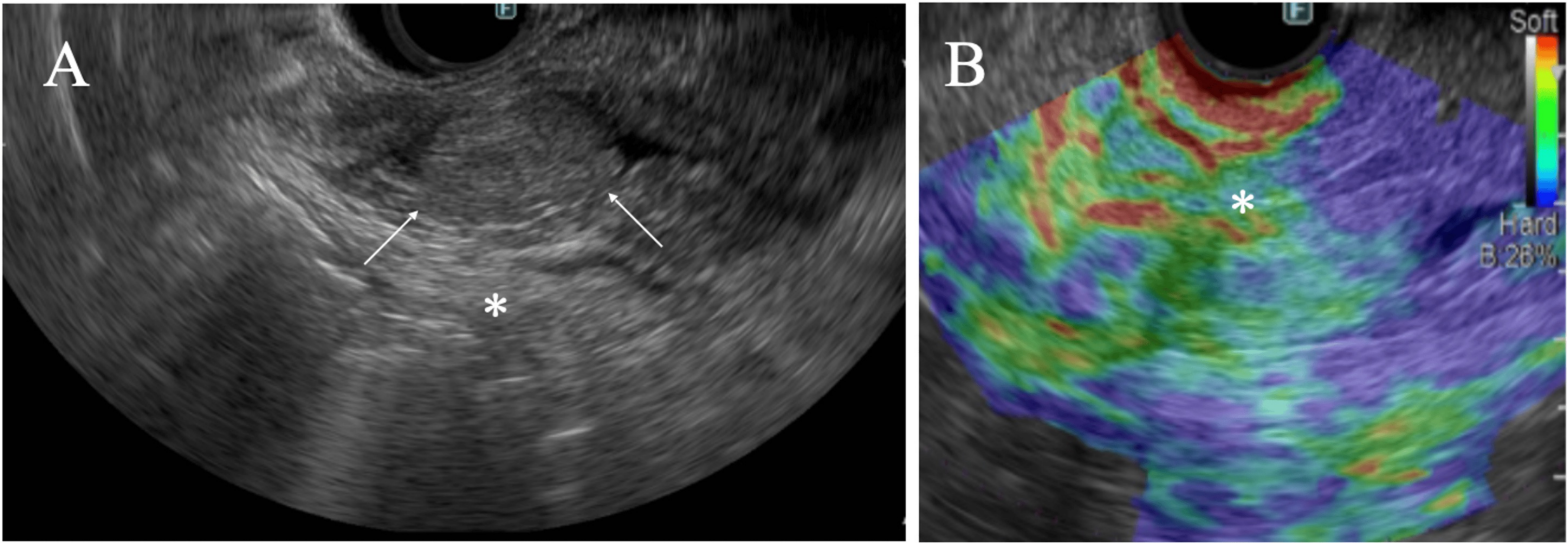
Endoscopic ultrasound (EUS) and elastography. (A) EUS showed a well-circumscribed mass (arrows) with internal high echoes and enhanced posterior echoes (asterisk). (B) Endoscopic elastography showed that the mass was expressed as greenish (asterisk).
